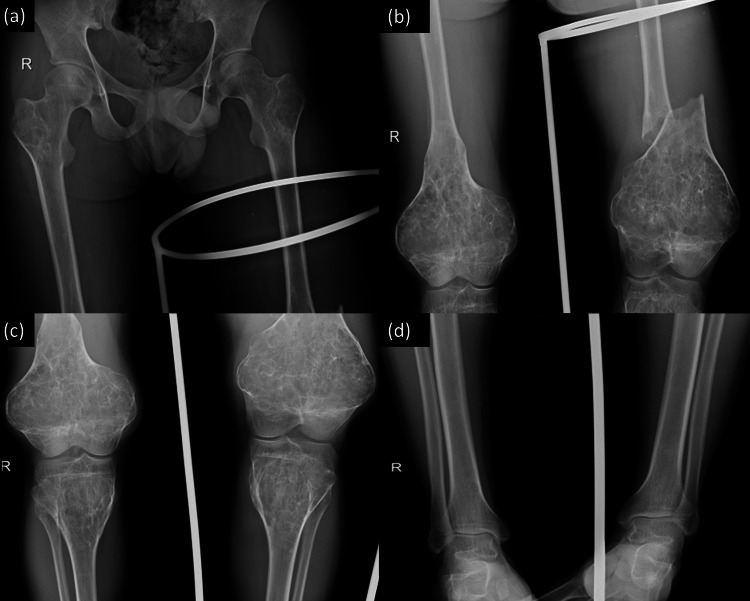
X-rays showing a loss of cortical continuity in the left distal femur and significant flask-like enlargements in the metaphyseal part of both distal femurs and proximal tibia, featuring numerous septations and thinned cortices.
